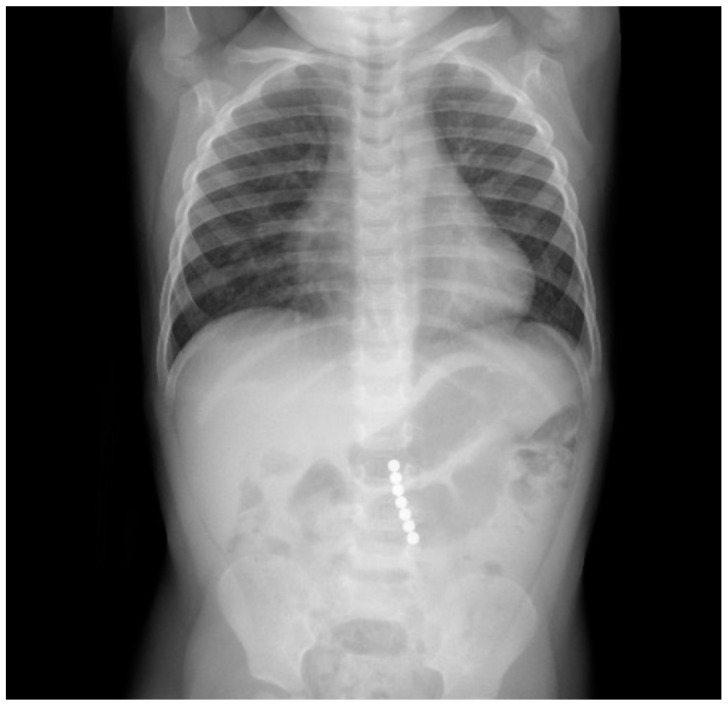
Conventional radiography showing the ingested magnets.
